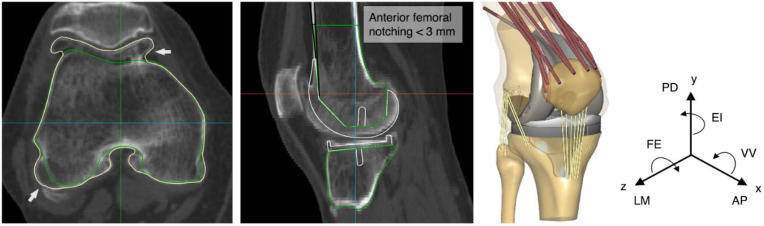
From left to right, axial view of the patient’s computed tomography (CT) scan indicating the osteophyte (white arrows, yellow line) and osteophyte-free femoral boundaries (green line); sagittal view of the mechanically aligning (MA) virtual planning; and the patient-specific musculoskeletal total knee arthroplasty (TKA) model. Nomenclature: AP, anterior-posterior; LM, lateral-medial; PD, proximal-distal; FE, flexion-extension; EI, external-internal; VV, varus-valgus.
